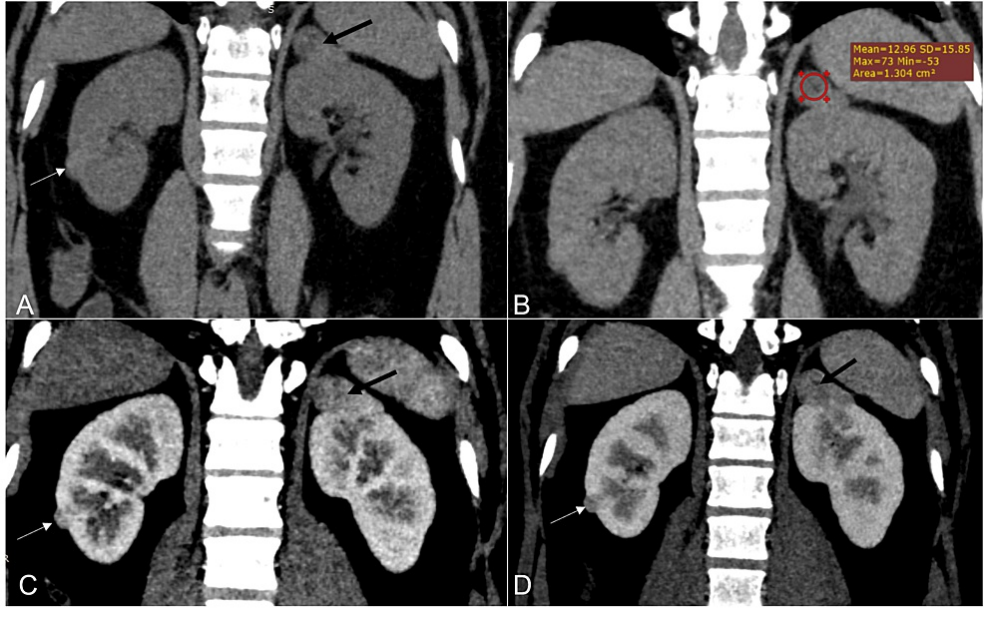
A 20-year-old male patient diagnosed with tuberous sclerosis Reformatted coronal NCCT (A & B), CECT corticomedullary phase (C), and nephrographic phase (D) images show multiple lesions in both kidneys (white and black arrows). The lesion in the left kidney upper pole shows fat attenuation with HU values of -53 HU in the ROI (B). Lesions in both kidneys show enhancement in the corticomedullary phase (C) and washout in the nephrographic phase (D), consistent with lipid-poor angiomyolipoma. This represents a Bosniak III lesion. NCCT: non-contrast-enhanced CT; CECT: contrast-enhanced CT; HU: Hounsfied unit; ROI: region of interest
